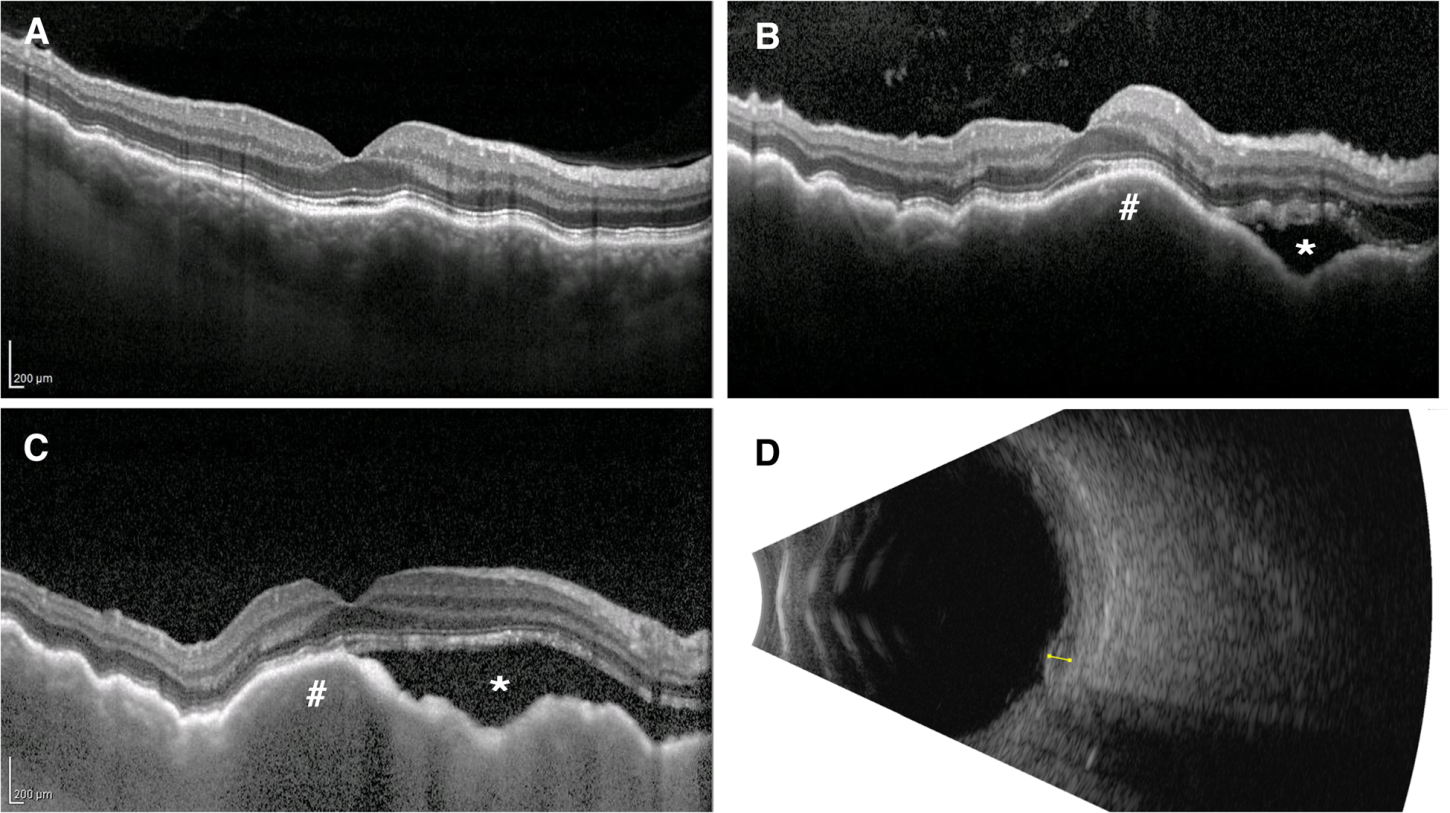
EDI-OCT of patient prior to treatment initiation. a) EDI-OCT at initial diagnosis of choroid folds; b) EDI-OCT following worsening of symptoms 11 months later; c) EDI-OCT on presentation to ophthalmology oncology clinic; d) ocular ultrasound with yellow line showing lesion depth of 1.5 mm. EDI-OCT = enhanced depth imaging optical coherence tomography’ * = subretinal fluid; # = choroidal lesion
